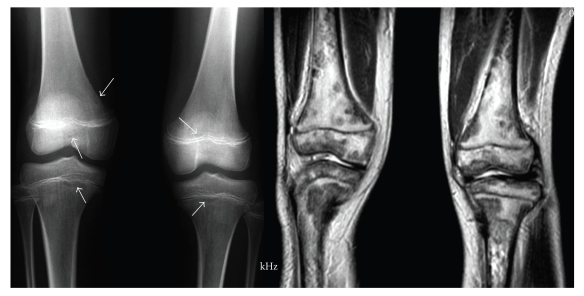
AP and MR image of both knees. AP film of both knees shows osteolytic lesions as
